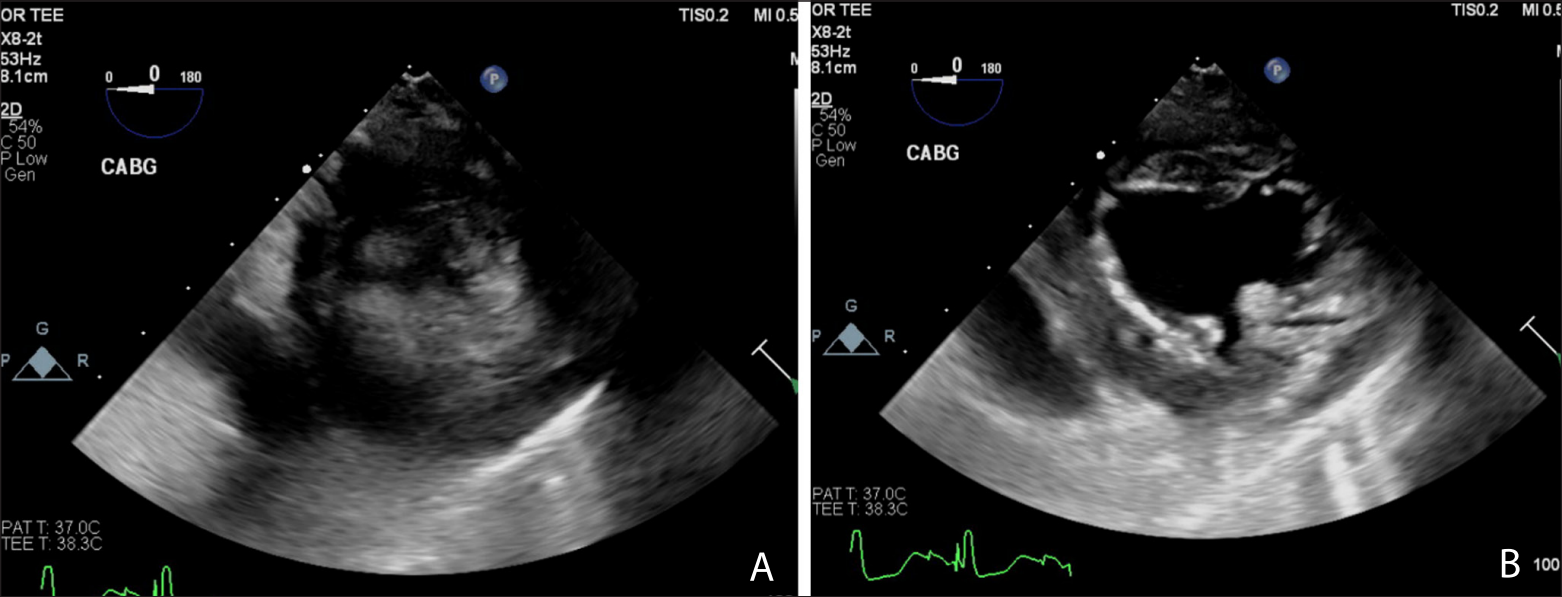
(A) Transgastric short axis transesophageal echocardiography (TEE) view of an underfilled left ventricle with normal function at end systole. This patient was hypotensive and responded to volume administration. (B) Transgastric short axis TEE view of an underfilled left ventricle with normal function at end diastole. Photo credit Kyle Buck, MD, Atrium Health Wake Forest Baptist Medical Center
